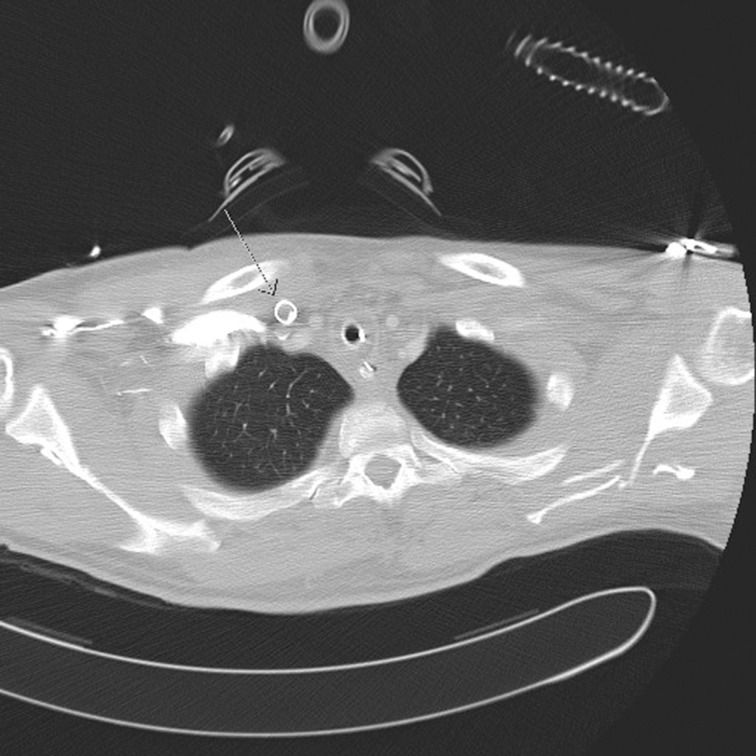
Computed tomography showing the entry of the cannula into the jugular vein with a partial thrombus in the lumen. However, there is no sign of a thrombus or adhesion around the cannula (arrow)
